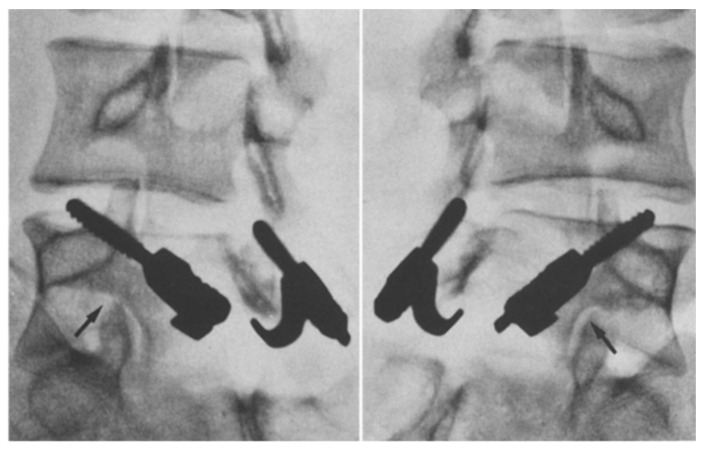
Radiographs showing solid fusion of L5 in the same patient one-year post-op [45]. The arrows depict the site of fusion. “Used with permission of Springer Nature BV, from Hefti, F.; Seelig, W.; Morscher, E. Repair of lumbar spondylolysis with a hook-screw. *Int. Orthop.* 1992, *16*, 81–85; permission conveyed through Copyright Clearance Center, Inc.”.
